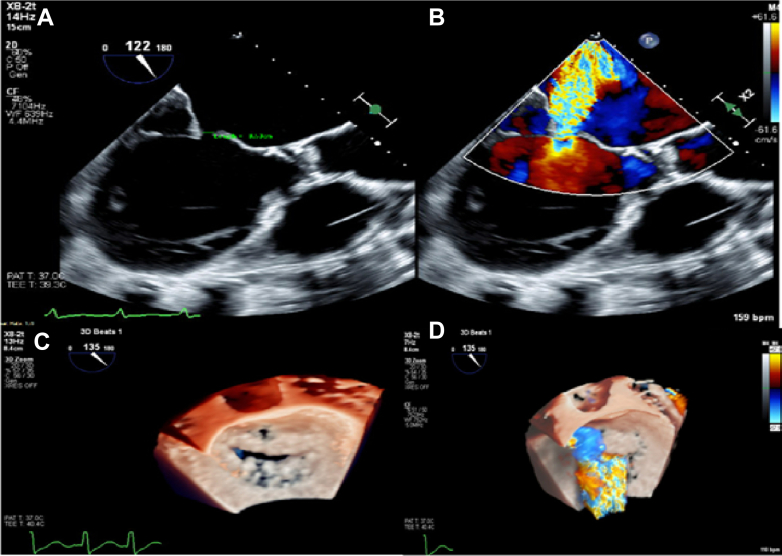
Transesophageal Echocardiography Images Confirming Severe Eccentric Mitral Regurgitation (A) Overriding anterior leaflets due to posterior leaflet tethering. (B) Color Doppler flow with severe regurgitation. (C) Three-dimensional image of coaptation gap during mitral valve closure in systole. (D) Three-dimensional color Doppler of mitral regurgitation in systole.
